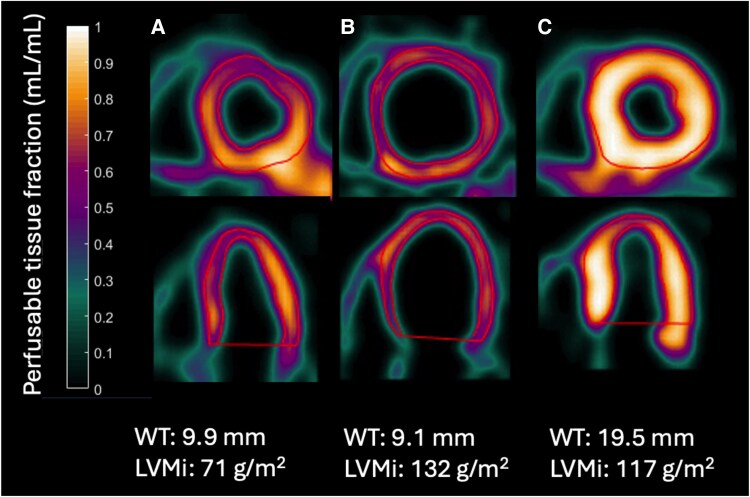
^15^O-water perfusable tissue fraction (PTF) images reoriented to cardiac short axis (upper panel) and horizontal long axis (lower panel) views showing patients with different cardiac geometry. Colour scale maximum is PTF = 1.0. (*A*) Male patient with normal PET findings and no known left ventricular hypertrophy. (*B*) Female patient with dilated cardiomyopathy. (*C*) male patient with cardiac amyloidosis and left ventricular hypertrophy confirmed by echocardiography. WT, interventricular septal wall thickness; LVMi, left ventricular mass index.
